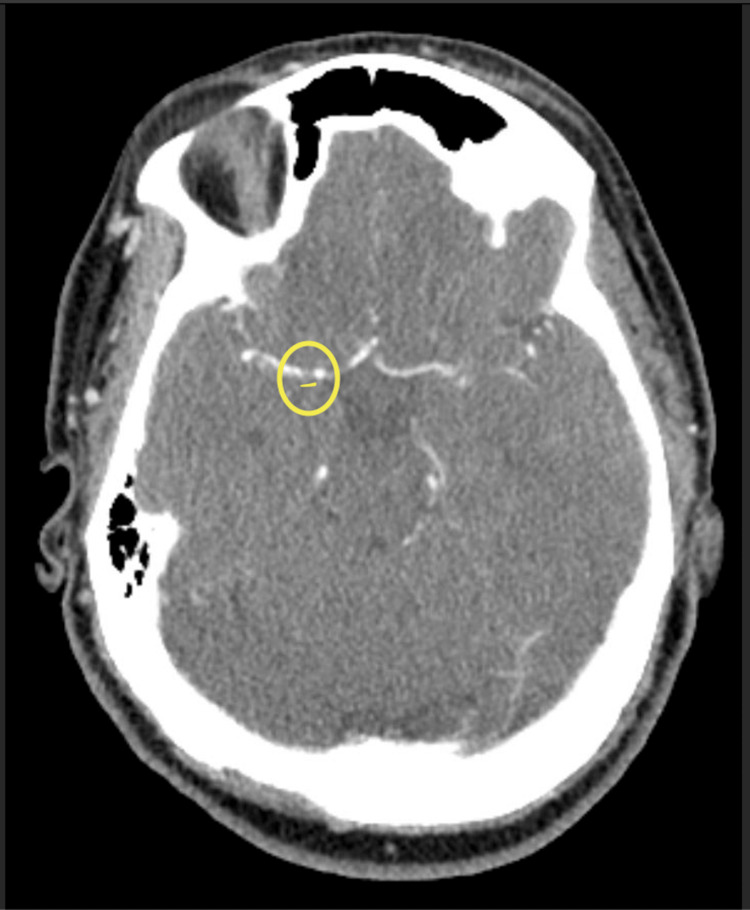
Axial computed tomography angiography (CTA) The circled region shows a short-segment stenosis of the proximal right M1 segment.
